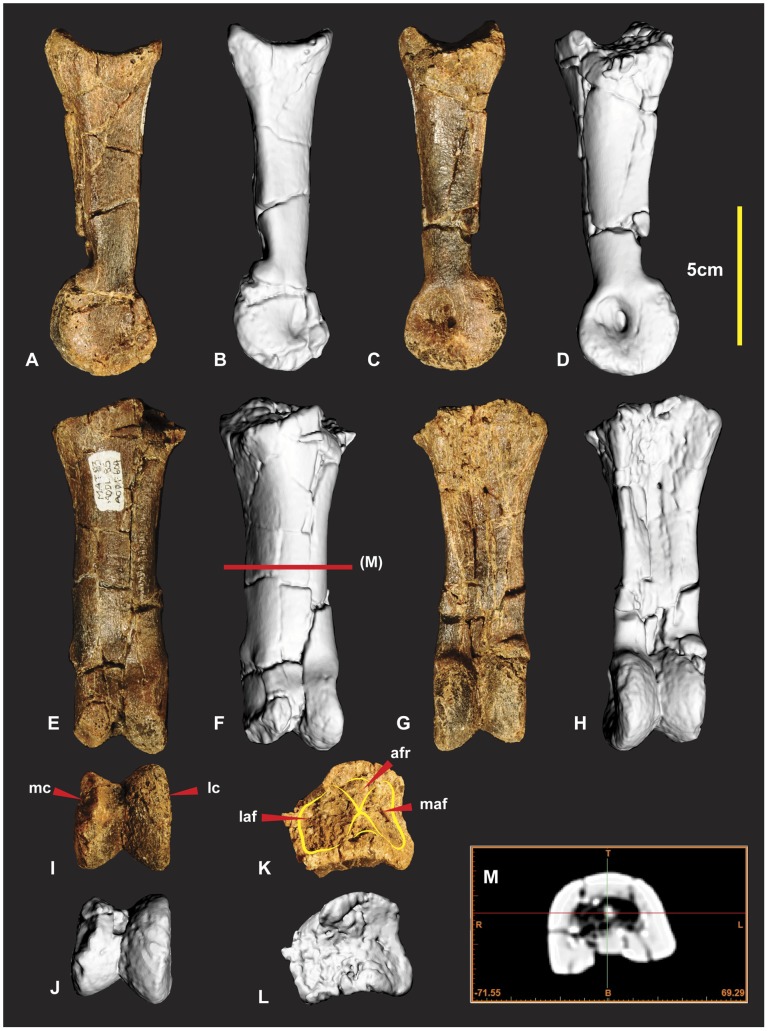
Left manual phalanx I-1. Left manual phalanx I-1 in medial (A & B), lateral (C & D), dorsal (E & F), ventral (G & H), distal (I & K), proximal (K & L) views, mid shaft CT image (M). *Abbreviations:* afr, articular facet ridge; laf, lateral articular facet; lc lateral condyle; maf, medial articular facet; mc, medial condyle.
